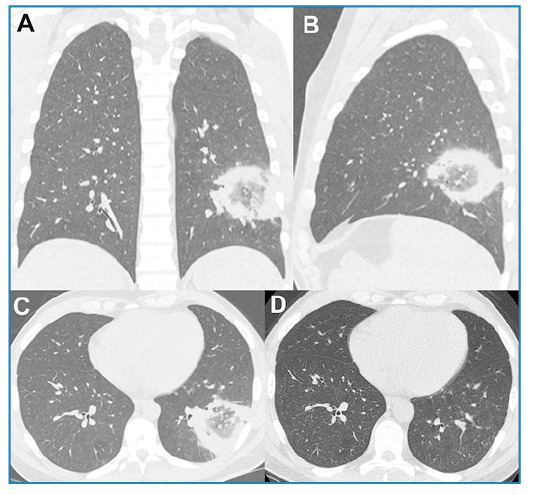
Chest computed tomography (CT) images with coronal (A), sagittal (B), and axial (C) reconstruction showing a rounded area of ground-glass opacity surrounded by a complete ring of consolidation in the left lower lobe, compatible with the reversed halo sign. Control CT performed three weeks after the initial examination (D) revealed complete lesion regression.
